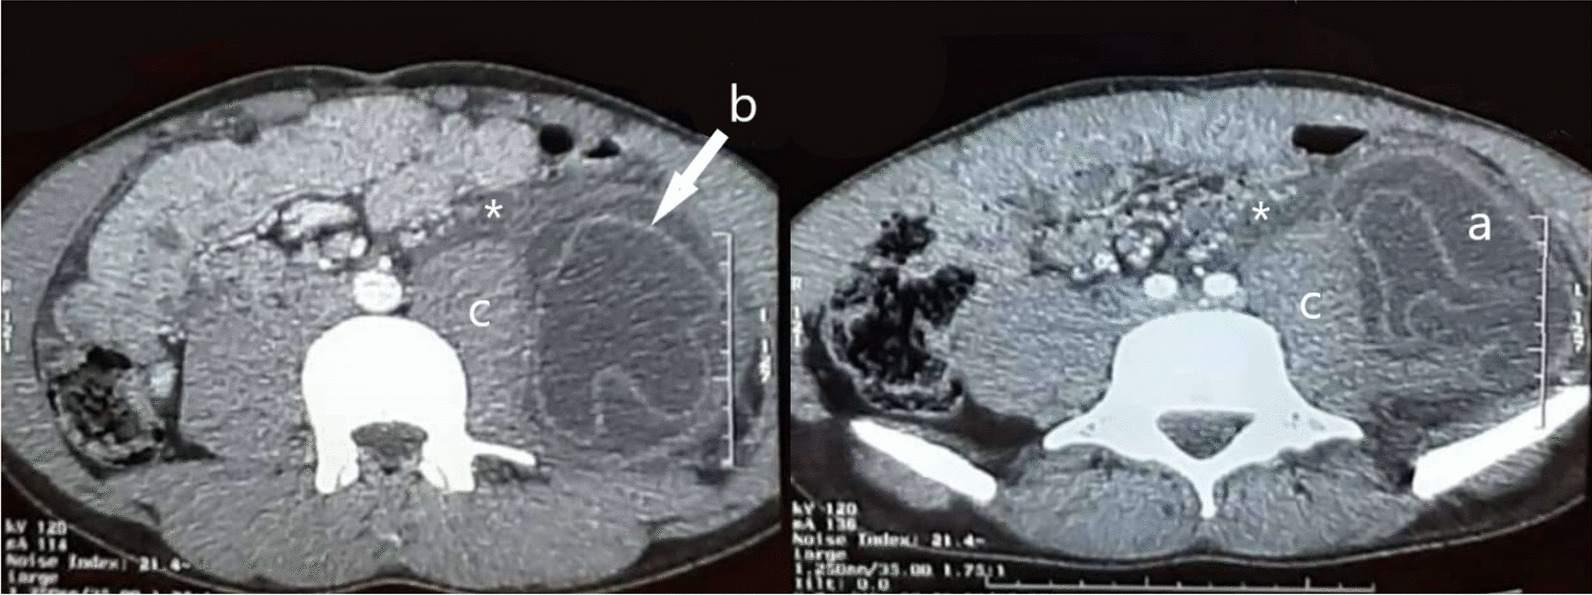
Computed tomography scan image showing hydatid cyst (a) with detached membrane b developed in the retroperitoneal space, adjacent to the psoas muscle c and associated to free peritoneal fluid*
